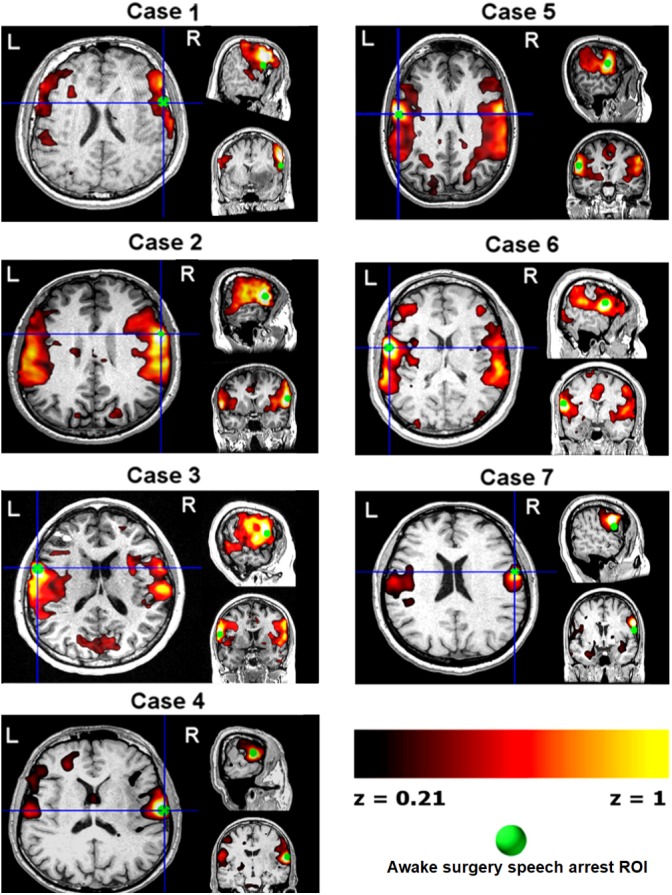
Single-subject pre-surgical resting state functional MRI connectivity networks (*z* > 0.21, hot color scale) using as seed each patient’s speech arrest location (green ROIs, Table 2 and Figure 3) obtained during awake surgery. Data are shown in each subject’s space. L/R, left/right brain hemispheres.
